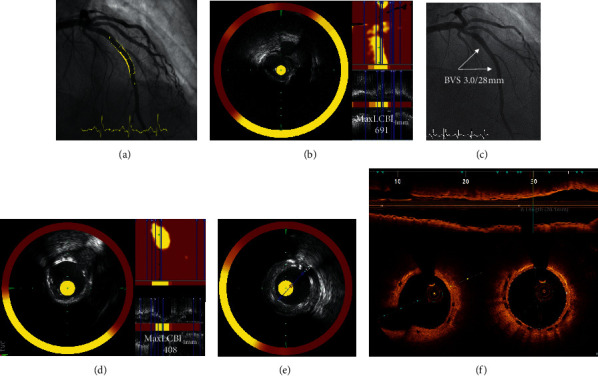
Example of persistently high maxLCBI_4 mm_ after BVS implantation. (a) Baseline angiography showing a moderate lesion in the mid-left anterior descending coronary artery. (b) Baseline NIRS showing a calcified NC-LRP. (c) Angiography of the BVS implantation. (d) Follow-up NIRS showing a persistently high maxLCBI_4 mm_ at the site of the original NC-LRP. (e) IVUS demonstrating good expansion of the scaffold in the calcified plaque. (f) OCT showing a good result of the BVS at follow-up with an appropriate expansion, apposition, and endothelialization. BVS = bioresorbable vascular scaffold; NIRS = near-infrared spectroscopy; NC-LRP = nonculprit lipid-rich plaque; IVUS = intravascular ultrasound; OCT = optical coherence tomography; and maxLCBI_4 mm_ = maximum lipid-core burden index in a 4 mm length of the artery.
